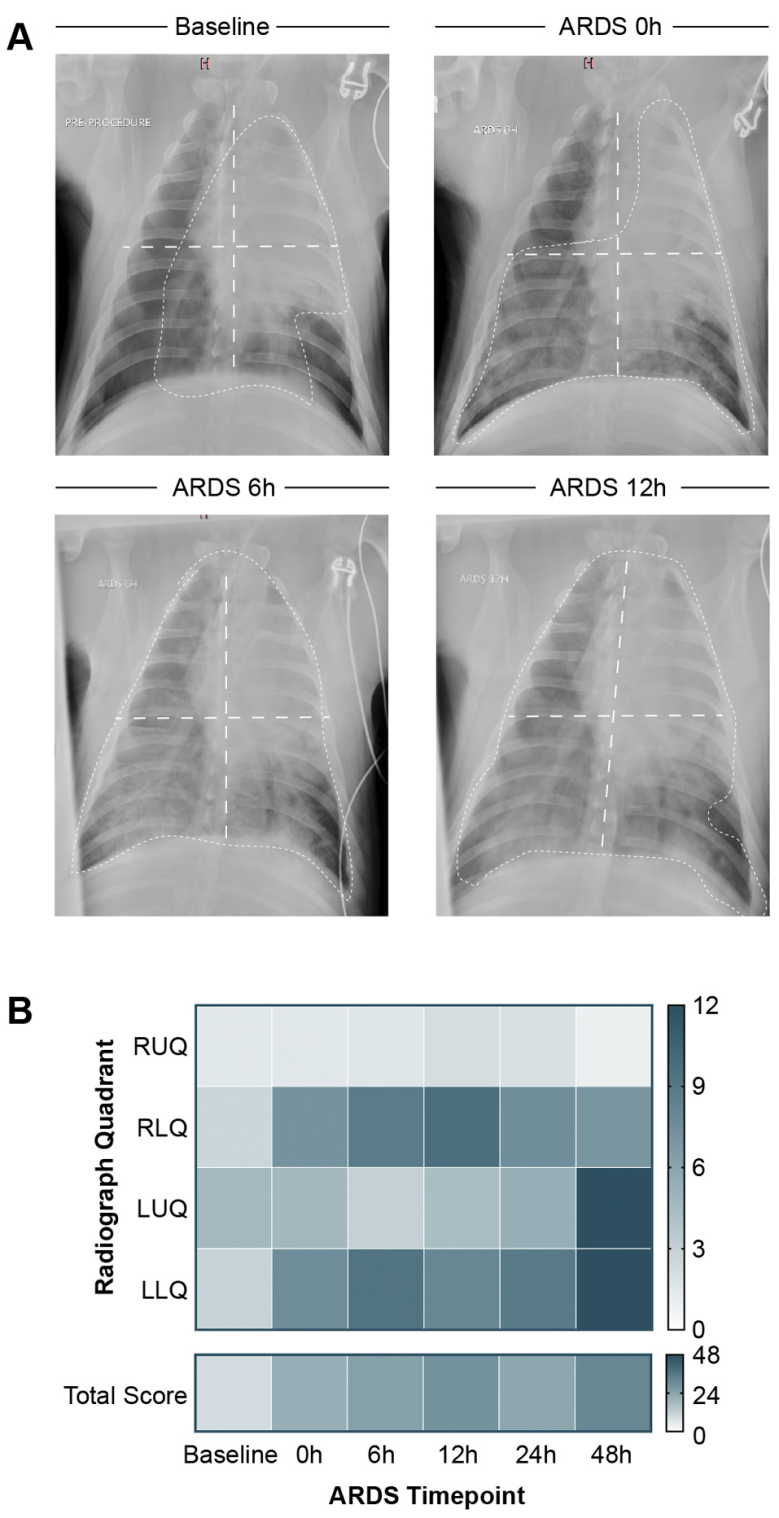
Radiographic progression of ARDS. (A) Representative chest X-rays from baseline up to ARDS 12 h demonstrate radiographically the progression of ARDS (long dashed white lines, approximate delineation of lung field quadrants; short dashed white lines, area of radiographic edema). (B) Median radiographic assessment of lung edema score per radiographic lung field quadrant and as total for ‘baseline’ lung (*n*=9), ARDS 0 h (*n*=7), ARDS 6 h (*n*=5), ARDS 12 h (*n*=3), ARDS 24 h (*n*=3), ARDS 48 h (*n*=1). RUQ, right upper quadrant; RLQ, right lower quadrant; LUQ, left upper quadrant, LLQ, left lower quadrant. Color scale from white (0) to dark blue (12, individual lung field quadrant; 48, total score).
